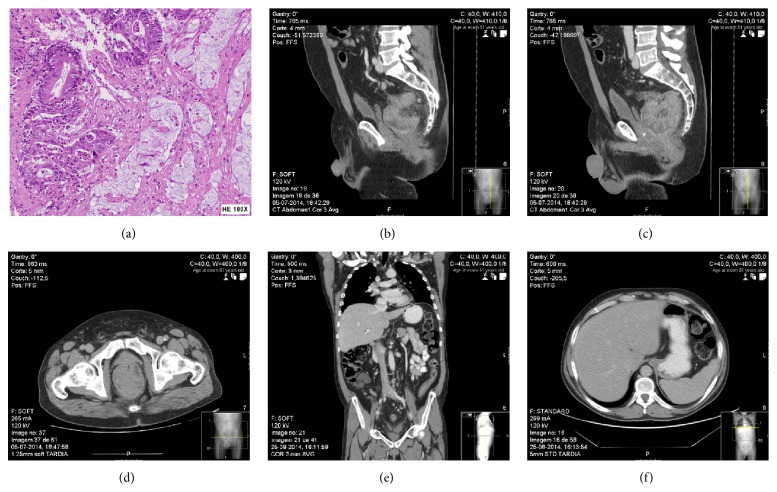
Diagnosis and staging. (a) Rectal biopsy mucinous adenocarcinoma; (b), (c), (d), (e), and (f) CT scan large tumor with 9 cm of longitudinal length and 7 cm of transverse diameter, plan between tumor and prostate not evident, multiple enlarged pelvic lymph nodes, and no evidence of inguinal nodal involvement or distant metastasis.
